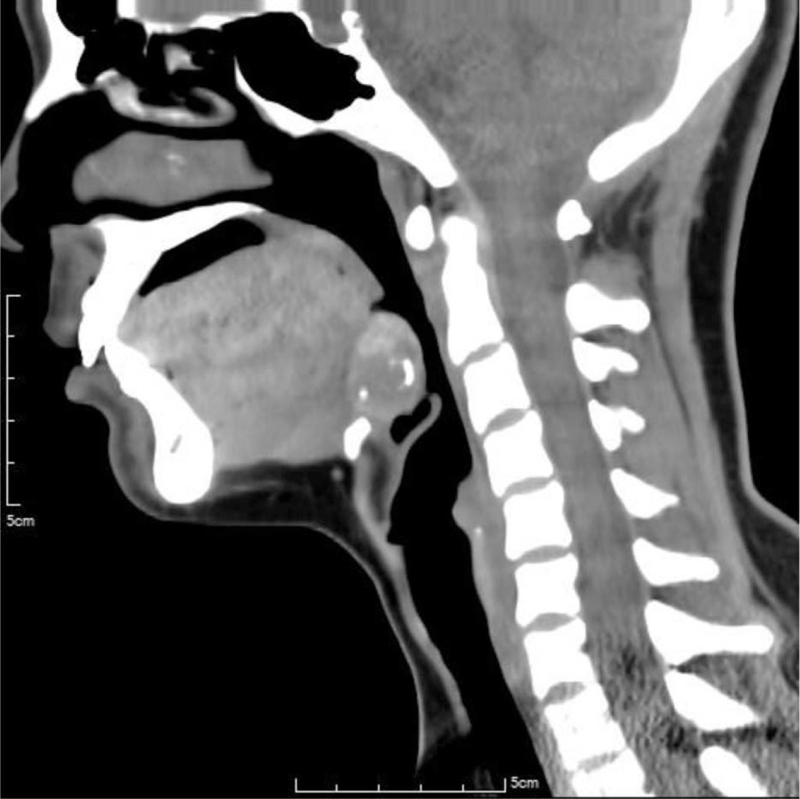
Non-contrast computed tomography scan of the neck showed a well-defined, mixed hyperdense and hypodense nodule, measuring 26 × 24 × 19 mm in the midline at the tongue base with focal ring calcification, suggestive of lingual thyroid. No thyroid gland was seen in the orthotopic location.
